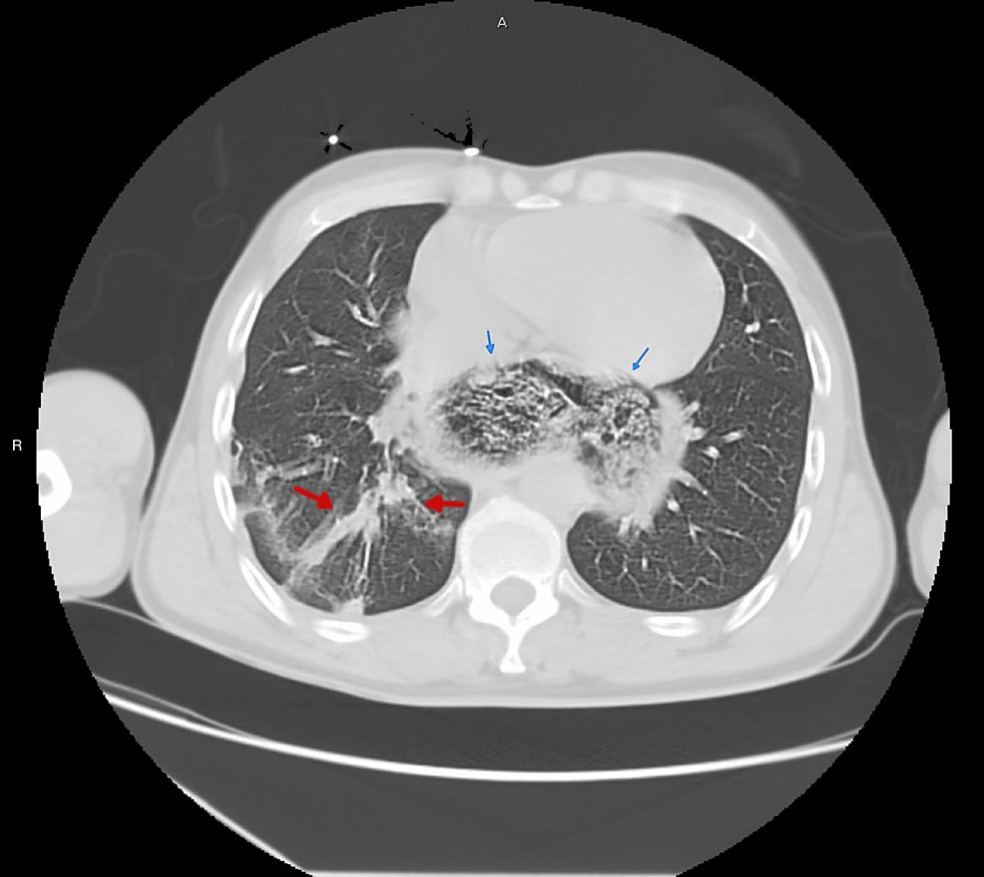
Lung enhancing transaxial CT scan of chest at the level of carina showing linear atelectasis (red arrows) and dilated esophagus with food debris and air (blue arrows).
